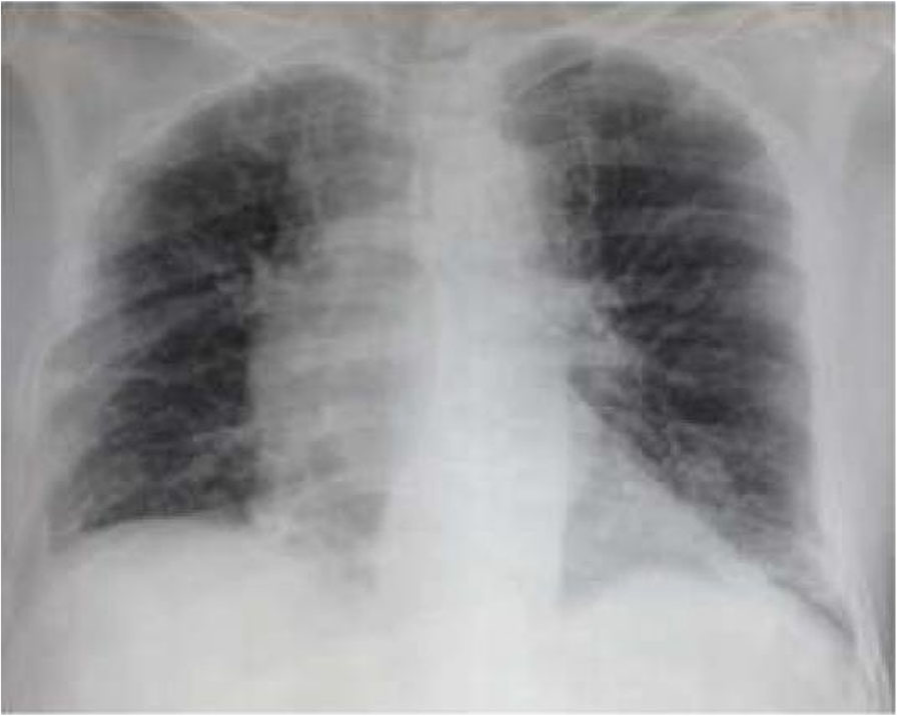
Chest X-ray showing bilateral interstitial infiltrates, predominantly in the bases
